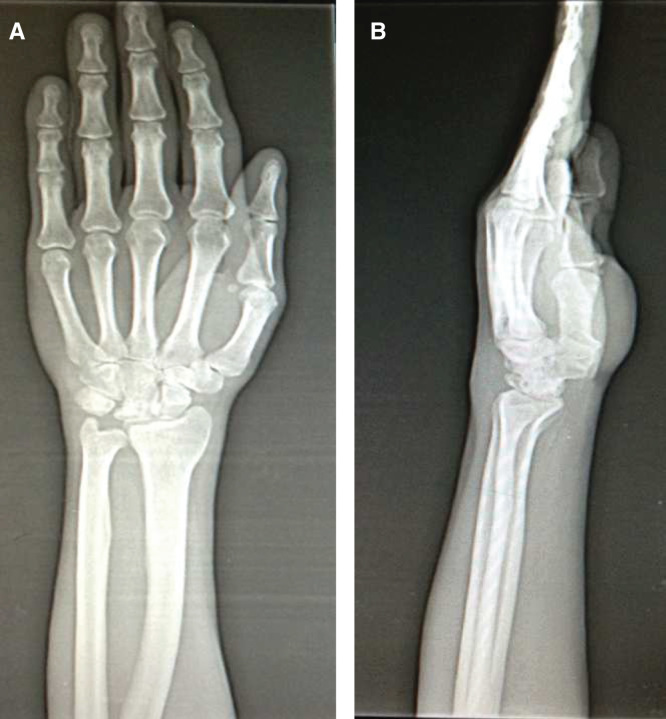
X-rays at second year of the operations showing the lunate to be in reduced position but has been collapsed (*a* and *b*).
